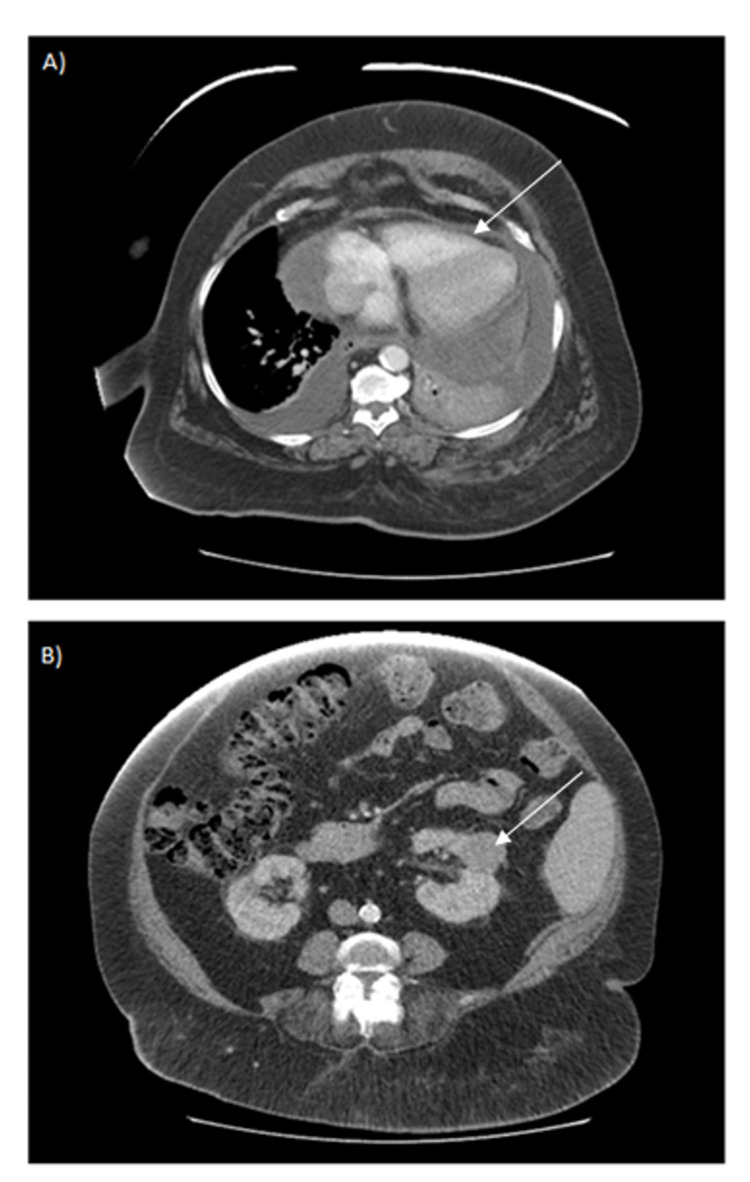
A) Chest CT scan showing a large pericardial effusion of heterogeneous density, and bilateral, left-predominant pleural effusion. B) Abdominal CT scan showing a solid mass in the interpolar cortex of the left kidney, measuring approximately 3.5 cm in diameter.
